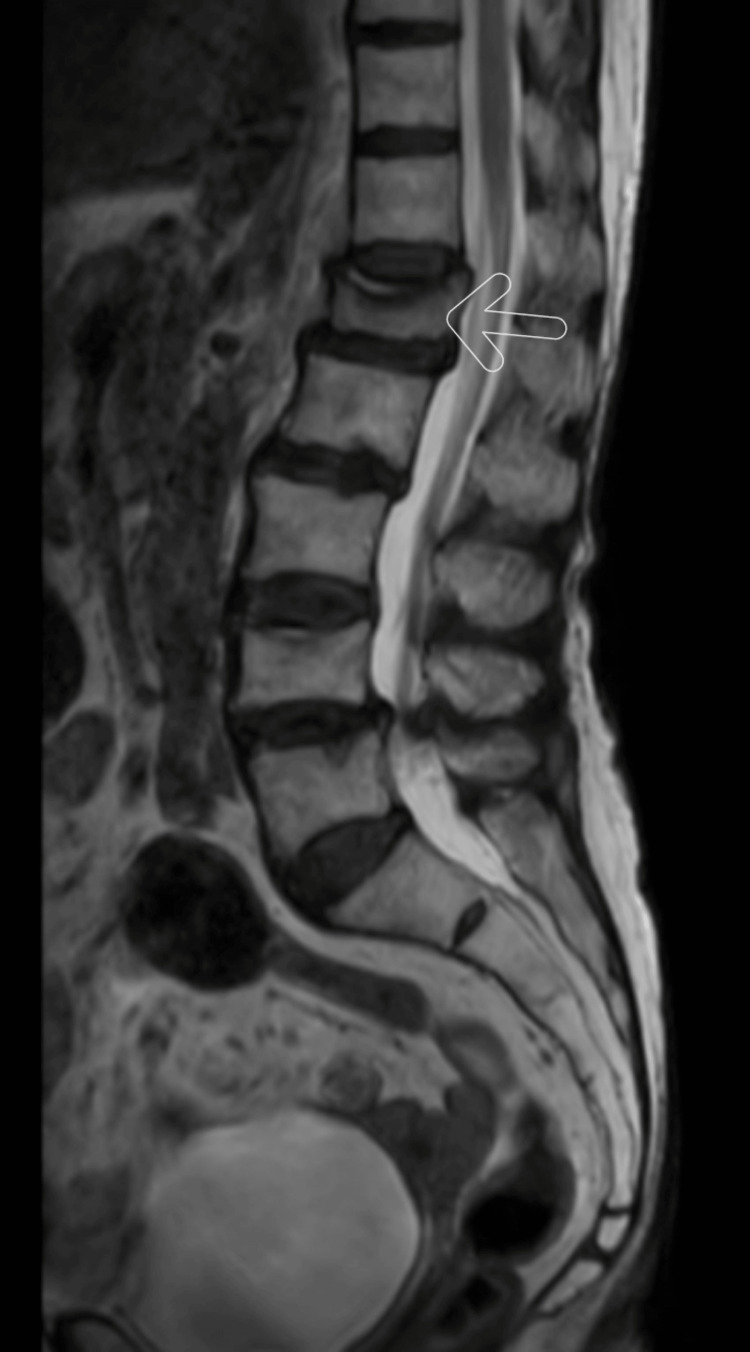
Sagittal MRI lumbar spine showing anterior wedge collapse of the L1 vertebra
